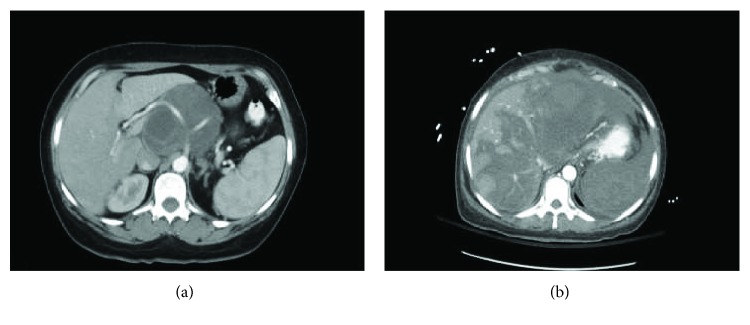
Abdominal CT scan showing (a) pancreatic mass involving the head of the pancreas, surrounding the celiac artery, in contact with the anterior aspect of the inferior vena cava, and (b) greatly enlarged pancreatic mass, extravasation of contrast from proximal splenic artery, and multiple heterogenous enhancements of the liver most likely indicating liver infarcts.
